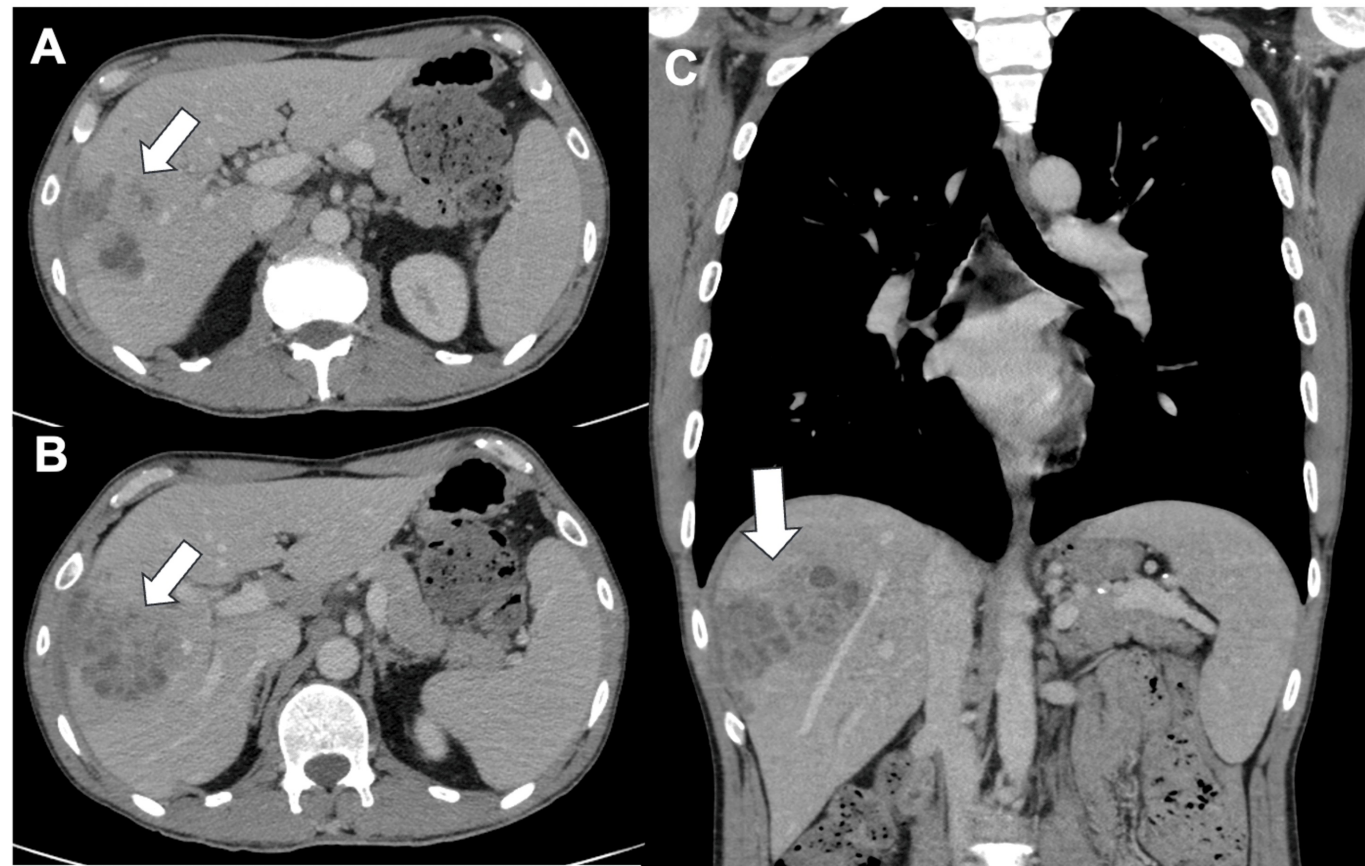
Abdominopelvic CT images A and B) Abdominal computed tomography (CT) axial images showing a heterogeneous hepatic collection with confluent microcystic areas and irregular margins (arrows); C) Coronal CT reconstruction demonstrating the extent of the hepatic collection, measuring approximately 8×7×6.2 cm (arrow).
